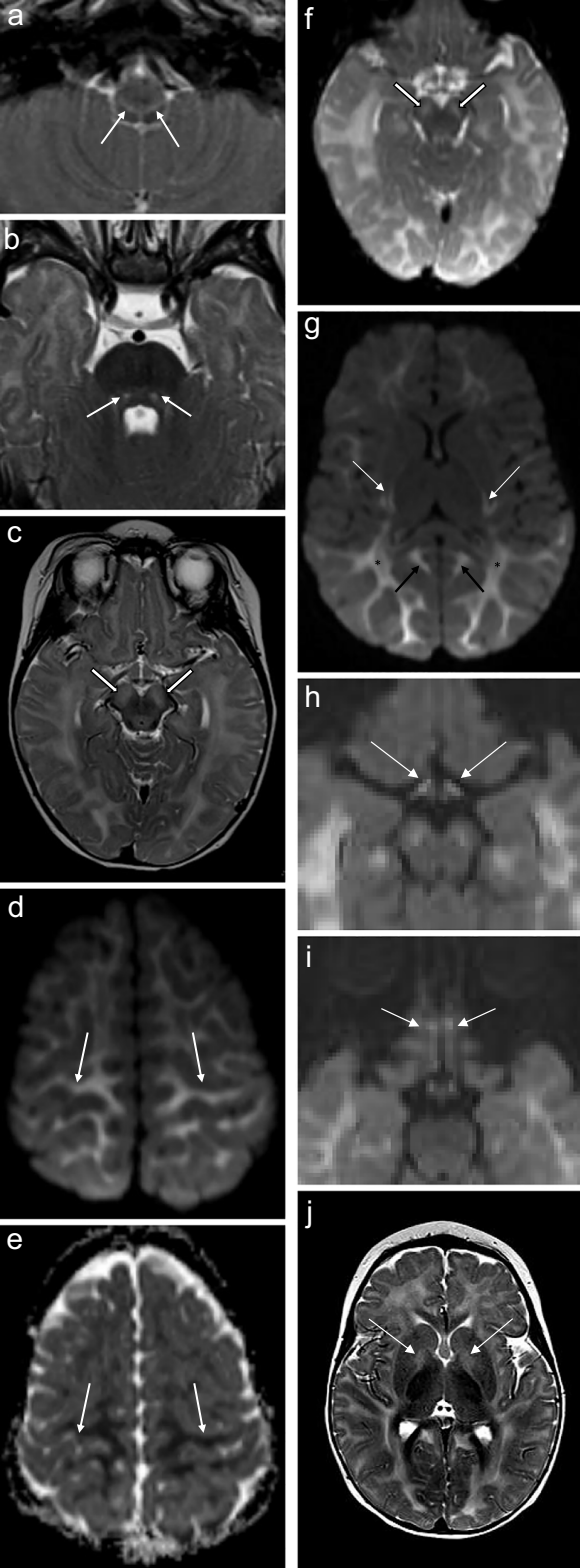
An 8-month-old girl with genetically confirmed *MPV17*-related mitochondrial depletion syndrome. a Axial T2-weighted image shows hyperintense signal in the reticular formation of the medulla (*arrows*). b Axial T2-weighted image shows hyperintense signal in the central tegmental tracts of the pons (*arrows*). c Axial T2-weighted image shows hyperintense signal in the cerebral peduncles of the midbrain (*arrows*). d Axial diffusion-weighted imaging and (e) apparent diffusion coefficient maps confirm restricted diffusion symmetrically present in the subperirolandic white matter (*arrows*). f Axial diffusion-weighted imaging shows restricted diffusion in the cerebral peduncles (*arrows*). g Axial diffusion-weighted imaging shows restricted diffusion present within the white matter (*asterisks*), posterior limbs of the internal capsules (*white arrows*) and cingulate gyri (*black arrows*). h* DWI *image shows restricted diffusion in the optic tracts and olfactory nerves (*arrows*) (i). j Axial T2-weighted image shows hyperintense signal in the medial globus pallidi (*arrows*)
